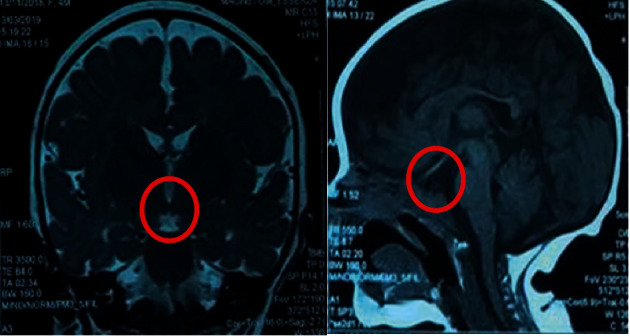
MRI of the head revealed a small anterior pituitary gland, an invisible stalk, and an ectopic posterior lobe.
